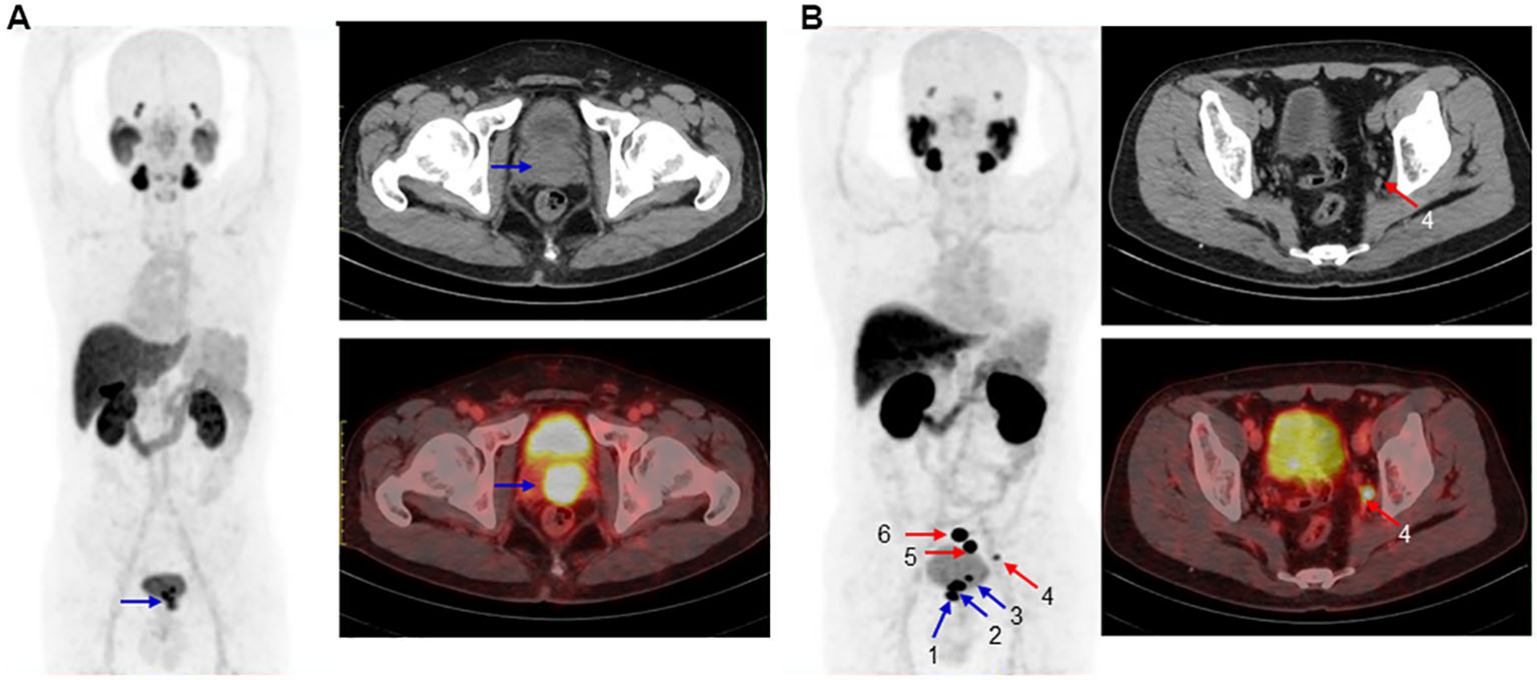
Maximum-intensity projections (left), CT image (top right) and PET/CT image (bottom right) of 2 patients at 1 h p.i.. (A). In a 57 years man of No.7 with PSA of 130.29 ng/mL and Gleason score of 4+3=7. The blue arrow indicated primary PCa (SUVmax: 13.5). (B). In a 67 years old patient No.4 with PSA of 48.01 ng/mL and Gleason score of 4+4=8. The blue and red arrows indicated primary PCa (primary 1, 2 and 3 with SUVmax of 28.7, 25.4 and 17) and multiple lymph node metastases (SUVmax rang: 10.4–149.4), respectively. CT and PET/CT imgaes showed the metastasis 4 with SUVmax of 10.4.
